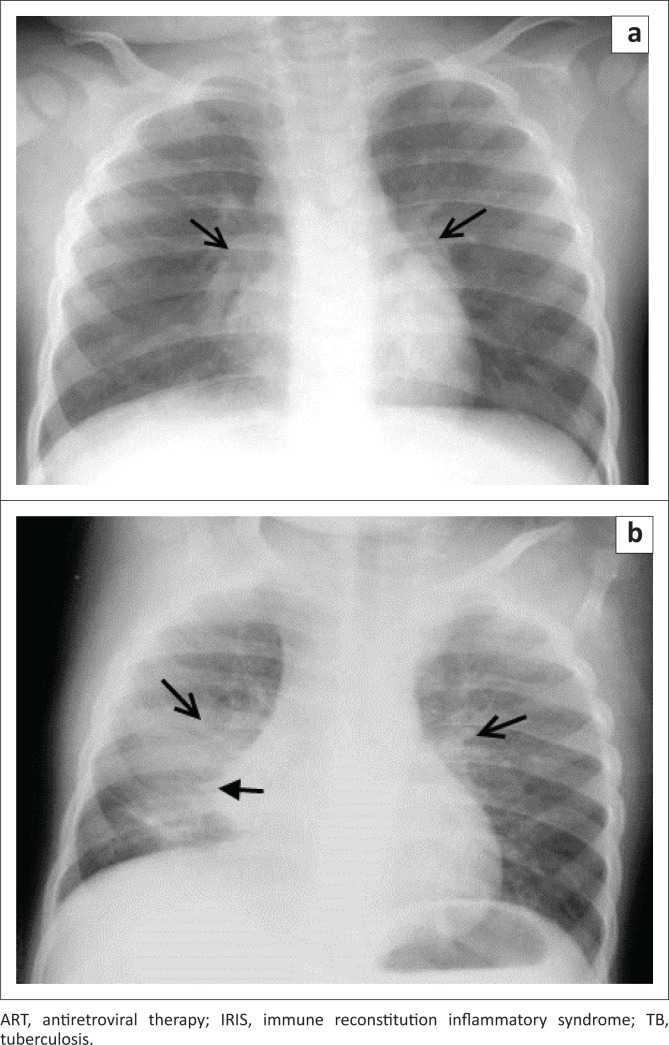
A 3-year-old HIV-infected girl initiated on ART 2 weeks after starting TB treatment. CD4 count was not available. Frontal chest radiograph taken at the time of ART initiation: (a) demonstrates bilateral hilar lymphadenopathy (open arrows). Chest radiograph taken 2 weeks after ART initiation; (b) demonstrates right middle lobe consolidation and collapse (closed arrow), with worsening hilar lymphadenopathy (open arrows). In combination with the clinical, radiological and laboratory findings, a diagnosis of paradoxical TB-IRIS was made.
